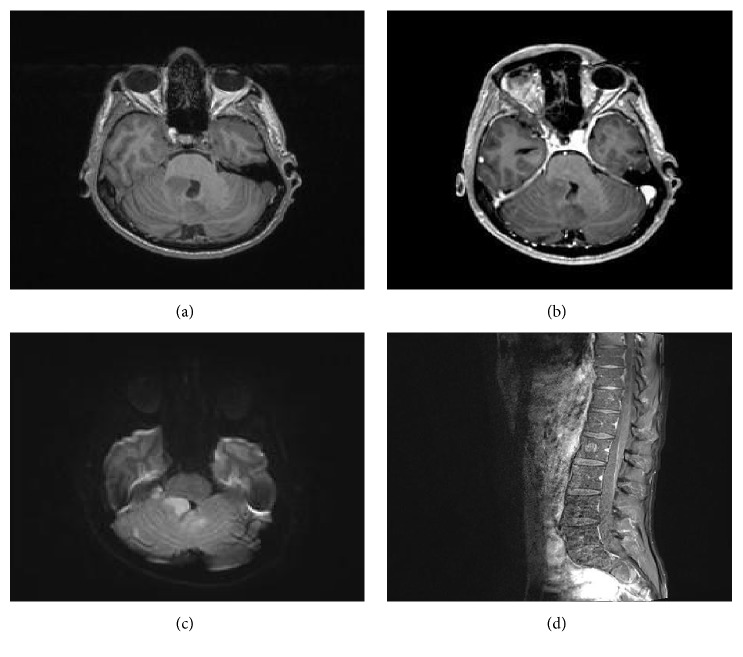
(a) Brain MRI T1 before contrast. (b) Brain MRI T1 after contrast. (c) FLAIR. (d) Spine MRI T1 after contrast.
